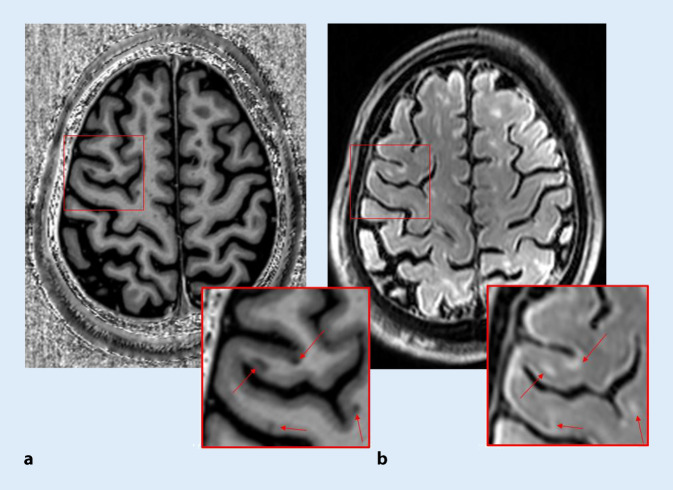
A number of cortical lesions in a patient with multiple sclerosis (39 years old, male) are well visualized on 7‑T MP2RAGE (a) and FLAIR (b)
